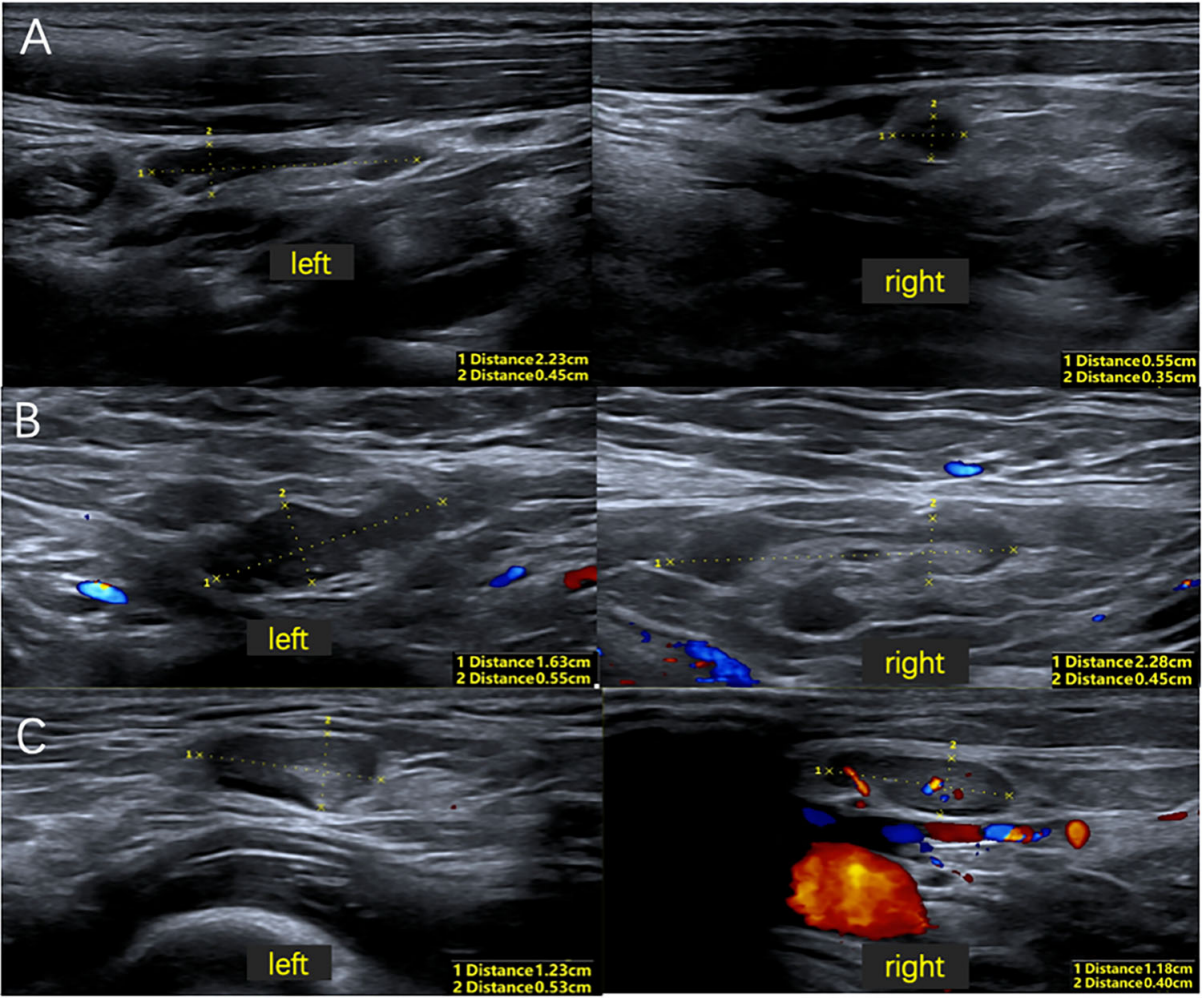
Ultrasound examination revealed enlargement of lymph nodes of bilateral cervical regions (A), axillary regions (B), and inguinal regions (C).
